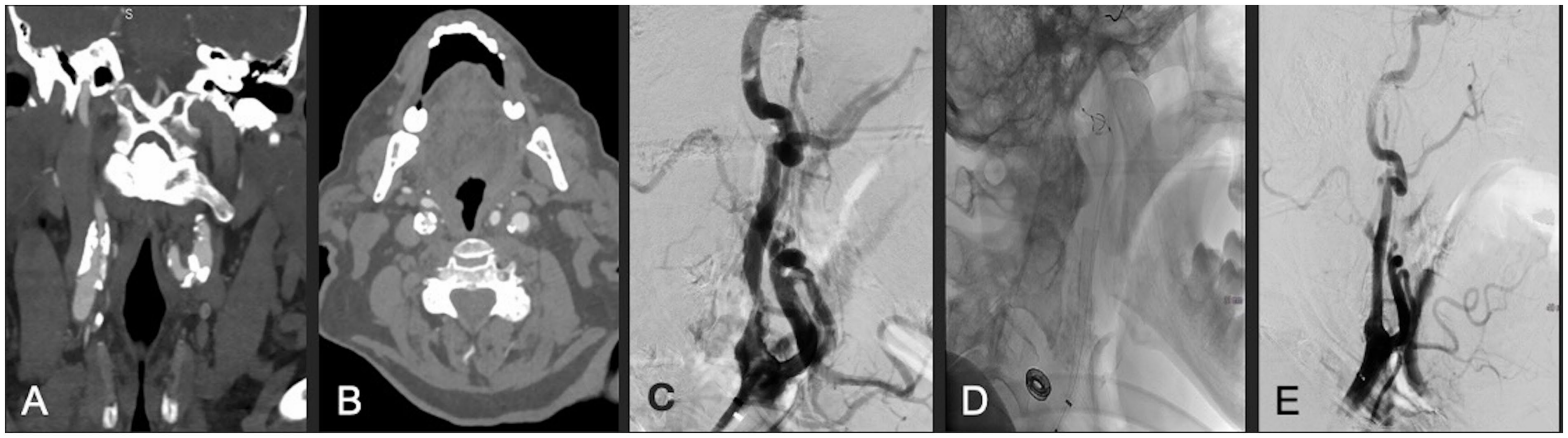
(A,B) Coronal and axial CTA demonstrating right ICA circumferential calcified stenosis. Catheter angiography demonstrating (C) severe ICA stenosis, (D) carotid stent and distal embolic protection device in place, and (E) significantly improved caliber and flow within right ICA following lithotripsy, stent and angioplasty.
